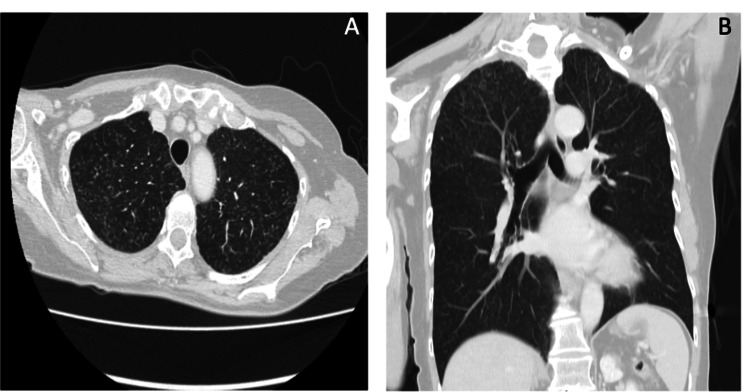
Chest CT images A and B: Chest CT images (axial and sagittal lung window). Multiple and bilateral ground-glass micronodules in a random distribution.
